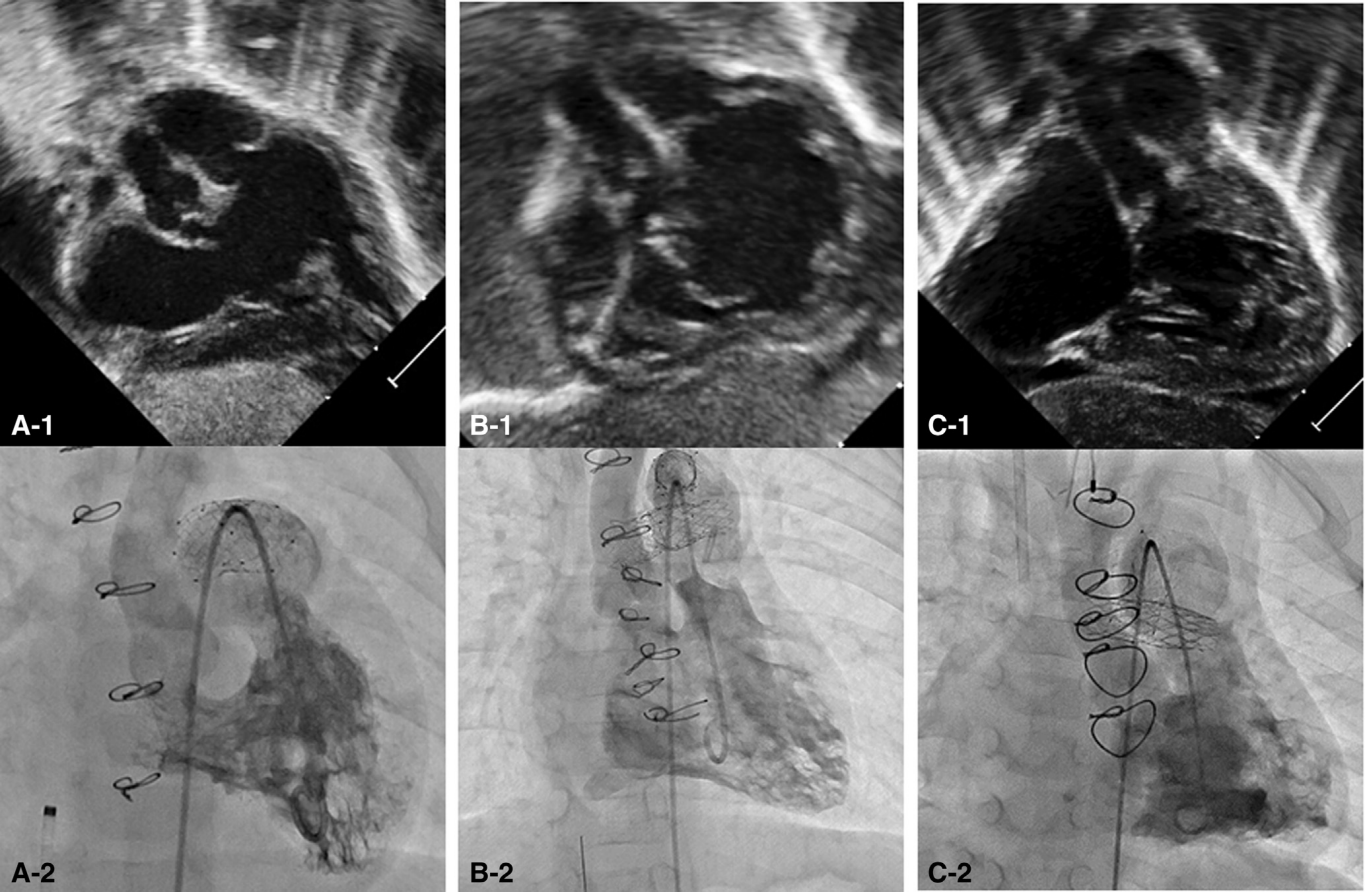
Initial subcostal echocardiograms (A-1, B-1, and C-1) and ventriculograms (A-2, B-2, and C-2) demonstrate the ventriculoarterial arrangements and relative sizes of the great vessel roots for patients 2 (double-outlet right ventricle), 3 (double-outlet right ventricle), and 4 (mitral atresia/ventricular septal defect), respectively. Adequacy of the native ascending aortic outflow is critical to demonstrate prior to attempt at the hybrid comprehensive stage II.
